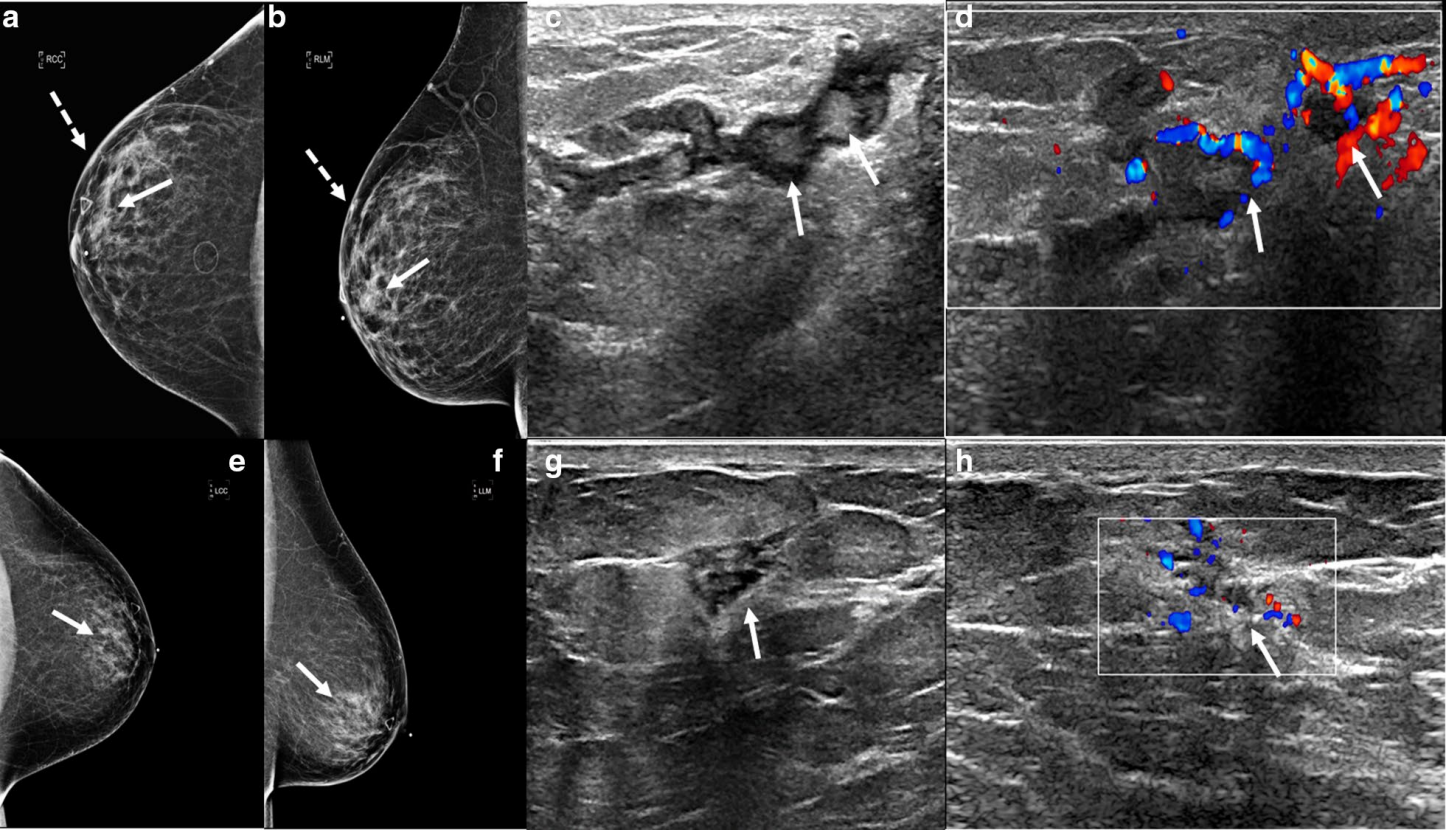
Lymphocytic mastopathy. A 37-year-old woman presented with right breast palpable retroareolar mass, swelling, erythema, and tenderness. Craniocaudal and lateral mammography (a, b) shows a focal asymmetry (solid arrows) and skin thickening (dashed arrows) associated with the triangular palpable marker in the lateral breast. Grayscale (c) and color Doppler breast ultrasound (d) reveals multiple retroareolar, vascular masses (arrows). Core needle biopsy showed acute and chronic inflammation and granulation tissue. The patient’s symptoms resolved within a month. The patient presented 2 years later with a contralateral palpable breast mass and nipple inversion. Craniocaudal and lateral mammographic views (e, f) reveal a focal asymmetry in the central breast associated with the palpable marker (arrows). Grayscale (c) and color Doppler (d) breast ultrasound reveals a vascular heterogeneous mass (arrows). Core needle biopsy revealed benign breast tissue with lymphoplasmacytic infiltrate. The patient’s symptoms improved over the following 6 months. She did not have a history of diabetes or other known immunologic condition
